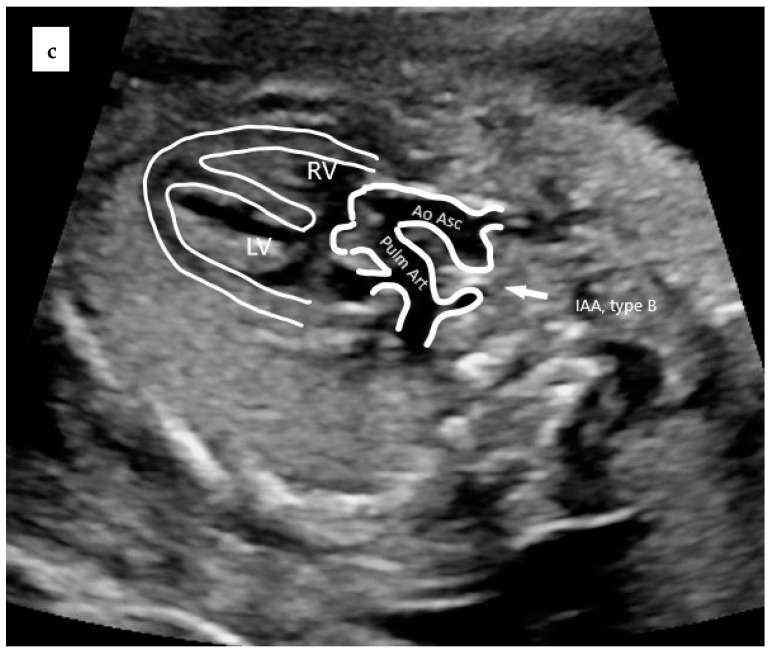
Prenatal echocardiography of TAC. (a) The five-chamber view showing the presence of a thickened truncal valve that overrides a large VSD (arrow); (b) The three vessels view showing two vessels: superior vena cava and truncal vessel (subtype A1): pulmonary trunk arising from the truncal vessel); (c) example of subtype A4 in which aorta and MPA arise from the common arterial trunk and interrupted aorta divides into brachiocephalic and left common carotid arteries (IAA Type B). TV = truncal vessel; VSD = ventricular septal defect; LV = left ventricle; RV = right ventricle; PT = pulmonary artery trunk, Ao Asc = ascending aorta; Pulm Art = pulmonary artery; IAA = interrupted aortic arch.
